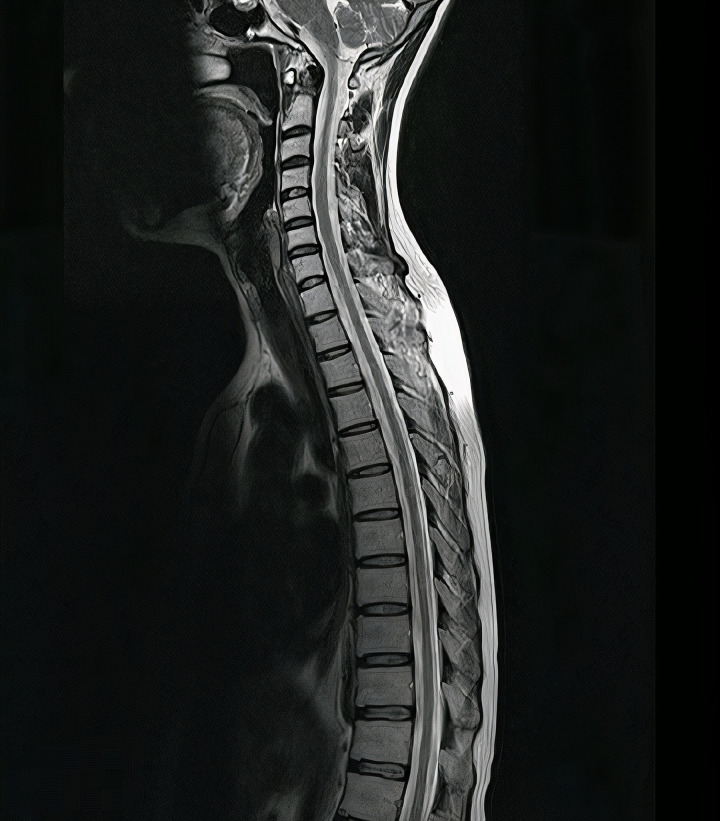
The sagittal view of T2-weighted Magnetic Resonance Imaging represented a mild decrease in spinal cord diameter without any other lesions at first admission.
